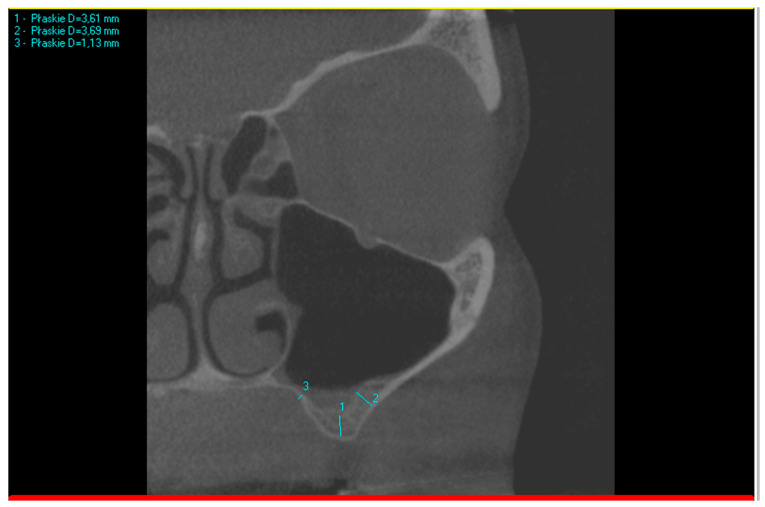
Example of three measurements taken on a CBCT scan. Measurement no. 1 is sub-sinus residual bone height, measurement no. 2 is the thickness of the lateral wall, and measurement no. 3 is the thickness of the palatal wall.
